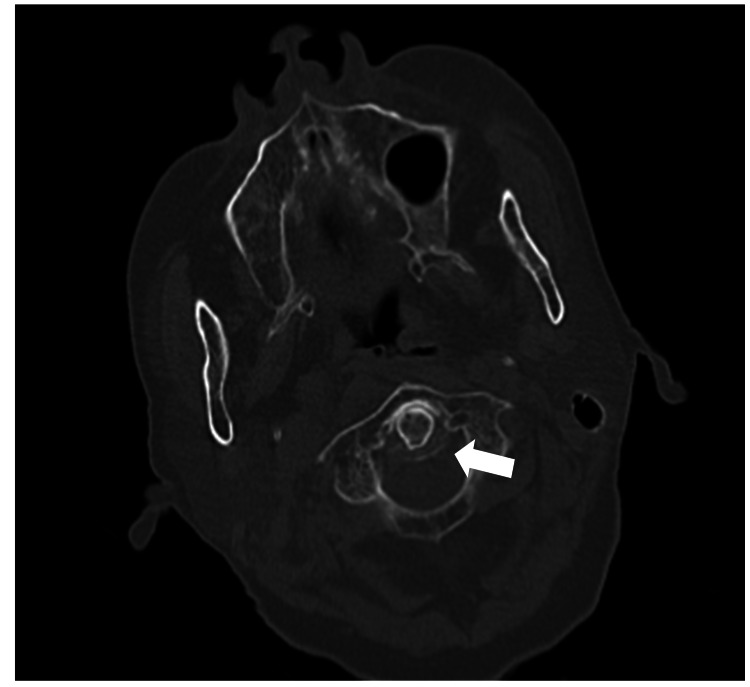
Cervical spine CT image Calcification around the second cervical odontoid process (white arrow).
